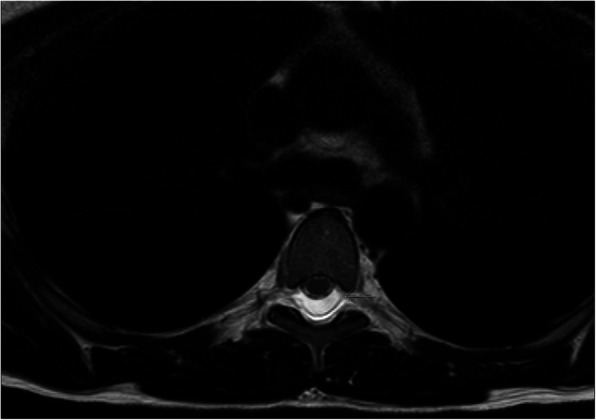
MRI (T2-weighted), TH5/TH6, horisontal: CSF surrounding nerve roots and dislocating the dural sac
